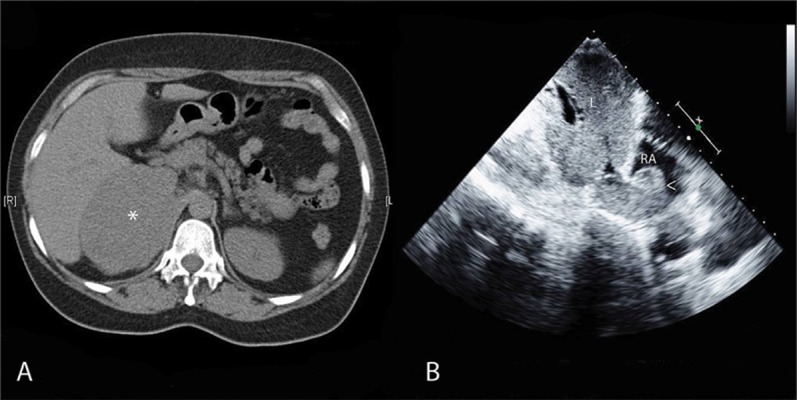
Computed Tomography Scan and Echocardiography Showing an Invasive Adrenocortical Carcinoma. A: Cross-sectional computed tomography scan showing a heterogeneous, solid mass measuring 9.3 × 7.4 cm in the right adrenal gland (asterisk), which was in close contact with the liver, the right kidney, and the inferior vena cava. B: Subcostal echocardiographic view showing infiltration of the inferior vena cava and a presumably metastatic mass in the right atrium (arrowhead). L: liver, RA: right atrium.
